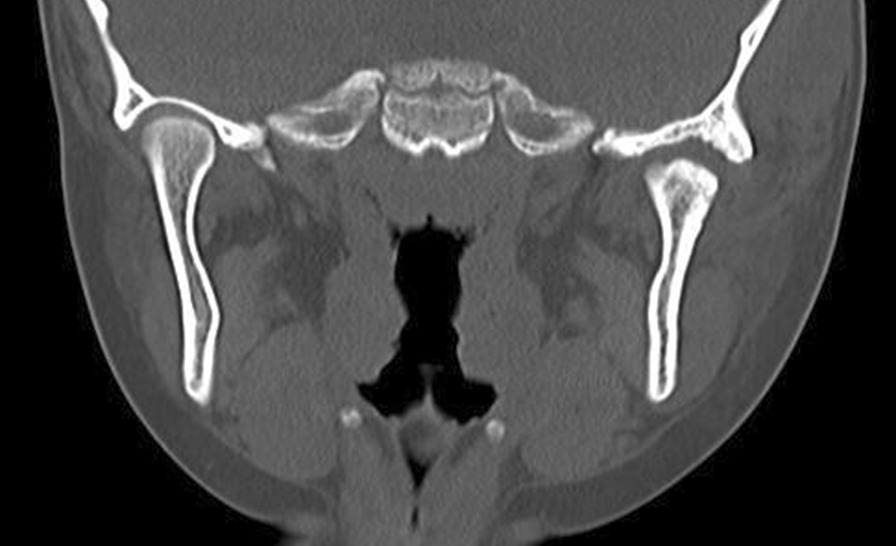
Postoperative CT demonstrating the structure of the patient’s joint cavity
